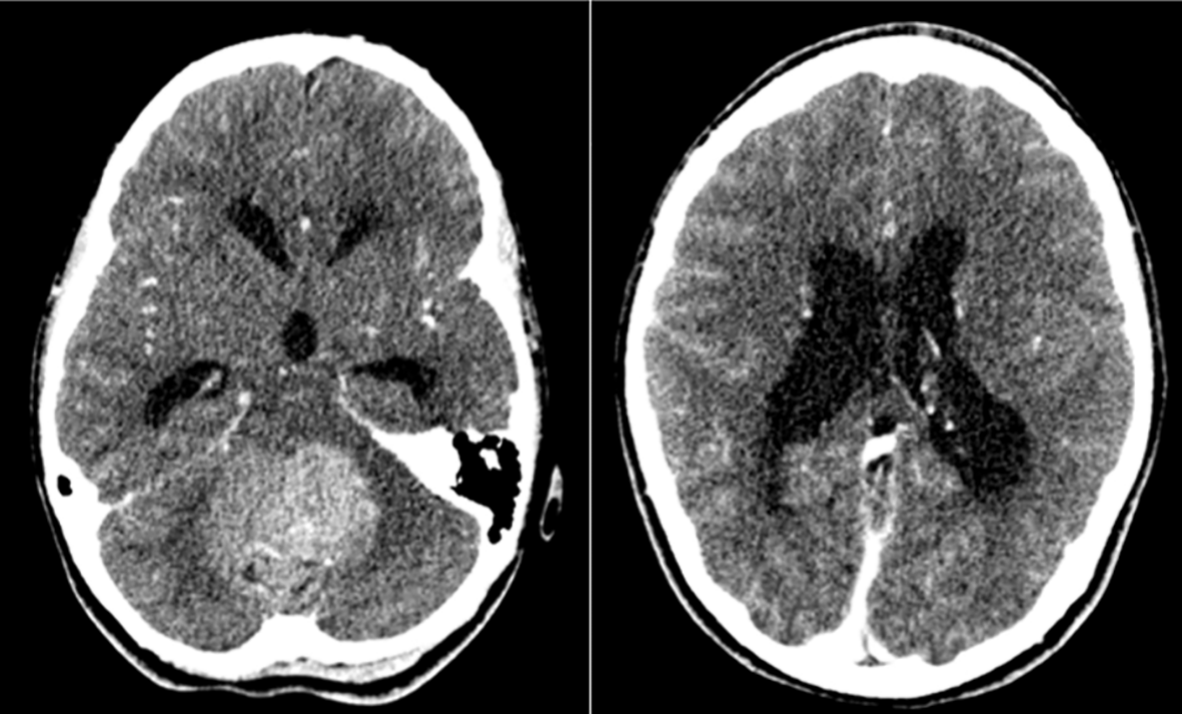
Axial post-contrast CT images demonstrating a large heterogenous mass arising from the posterior fossa (left image) and obstructive hydrocephalus secondary to the mass (right image) in our index case at time of initial presentation.
